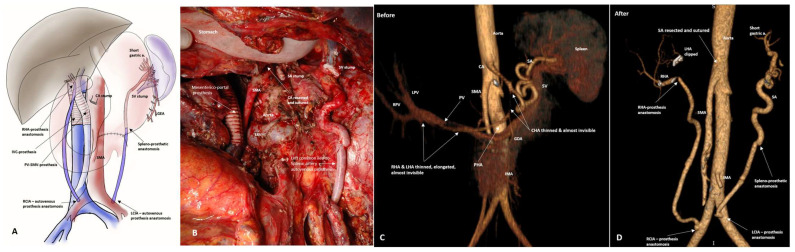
Removal of the recurrent gigantic MPNST in a 33-year-old lady with stomach- and spleen-preserving total pancreatectomy, right nephr- and adrenalectomy, and resection of the CA, SA, LGA, common, proper, left and right hepatic arteries, portal, splenic and inferior cava veins. Cava-caval and SMV-PV synthetic prostheses. Right common iliac to right hepatic artery and left common iliac to splenic artery autovenous prostheses. Spleen and the whole stomach were preserved after resection of the CA with the left and right gastric and RGEA due to revascularization of the SA remnant, which supplies the SGA-LGEA network. (A) A scheme of surgery after reconstruction of blood vessels and gastrointestinal tract: (B) An operating field after completion of the resection stage; (C,D) CTA before and after surgery, showing the new sources of the spleen and stomach blood supply.
